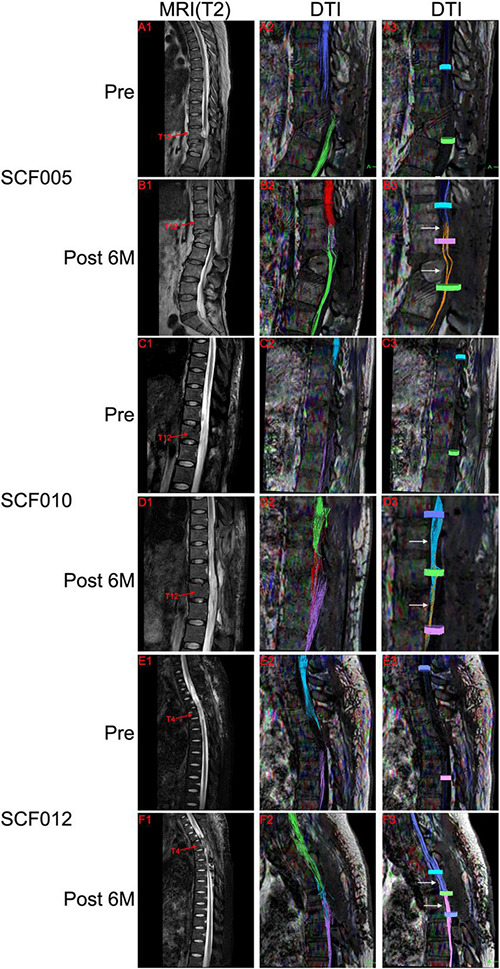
Representative neuroimages. Preoperative T2-weighted MRI scans showed spinal cord injury (A1,C1,E1), and DTI showed complete disruption of the spinal cord fibers (A2,A3,C2,C3,E2,E3). Postoperative T2-weighted MRI scans and DTI showed the autotransplanted sural nerve in the original spinal cord injury area (B1,B2,D1,D2,F1,F2). DTI showed restoration of neural connection at the two sites of transection (white arrows in B3,D3,F3). The colors of the fibers were automatically generated by the DTI system of MRI, with no practical significance. Pre, Preoperatively; Post 6M, 6 months postoperatively.
